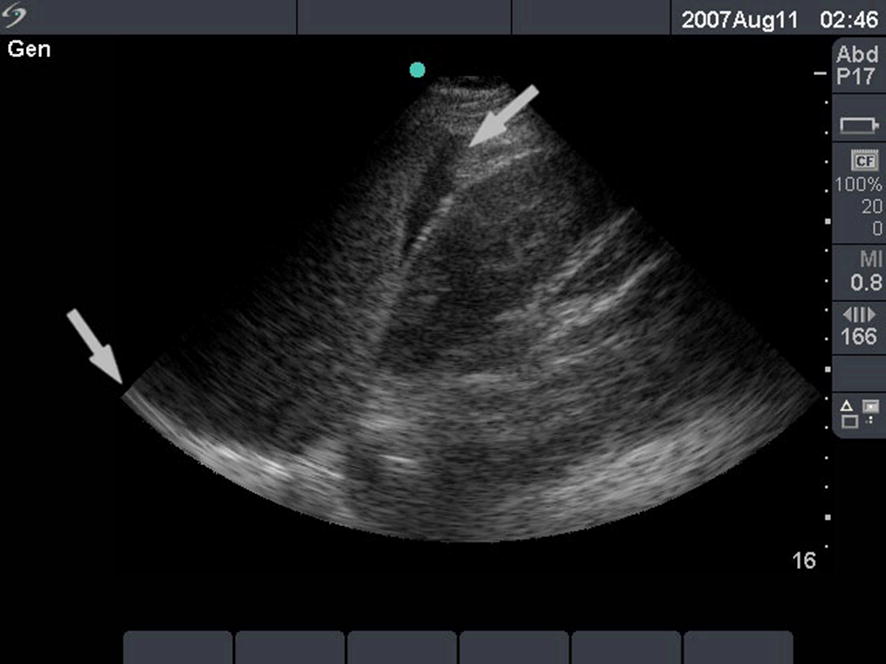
Hemoperitoneum at the splenorenal recess. An ultrasound image of the splenorenal recess (Koller’s pouch). A phased-array cardiac transducer was used with the abdominal settings. The left arrow indicates the diaphragm. The right arrow indicates the splenorenal recess with a hypoechoic collection between the spleen (left) and the left kidney (right). This is the image of free intraperitoneal fluid and is very suggestive for intraperitoneal hemorrhage when encountered in a trauma victim
